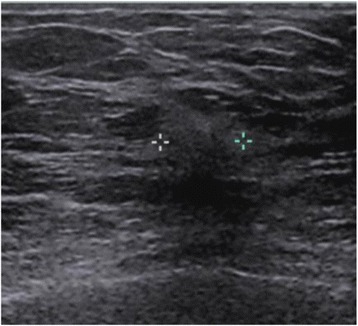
Grey-scale sonographic image of the same invasive lobular carcinoma shown in Fig. 1. Note the typical sonographic presentation with irregular margins, posterior acoustic shadowing, and disruption of normal fascial planes. Calipers are placed on the image to delineate margins of the mass
